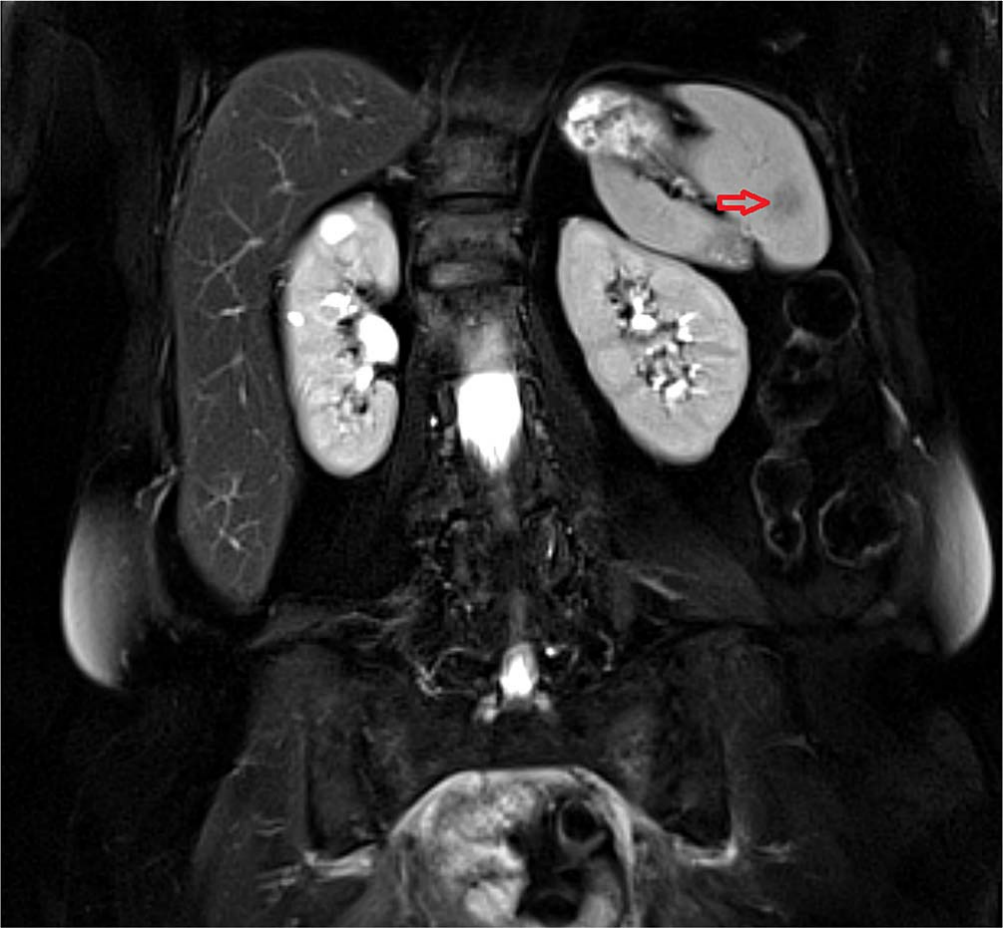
Coronal MRI plane demonstrating low signal intensity splenic lesion (arrow). T2-fat suppression image.
